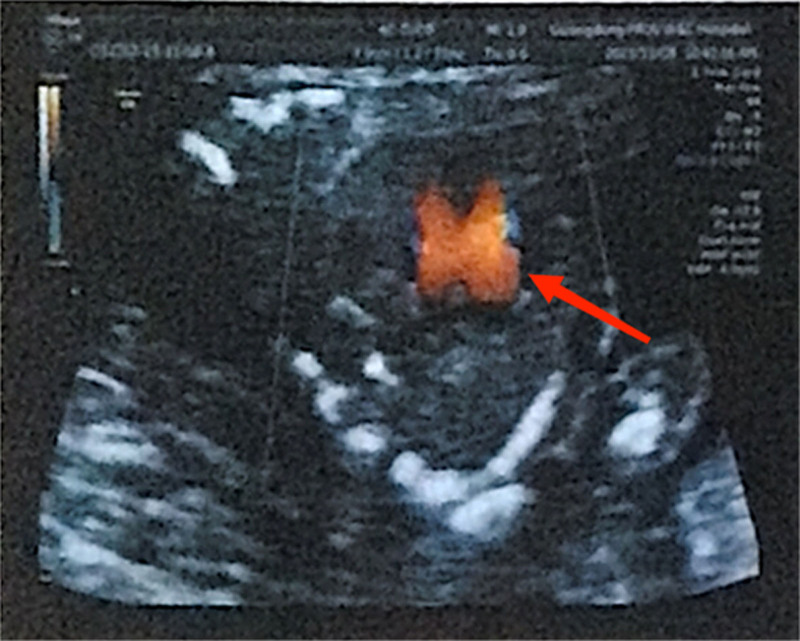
(November 2023) Ultrasound showed an intrauterine pregnancy with a single live birth.
